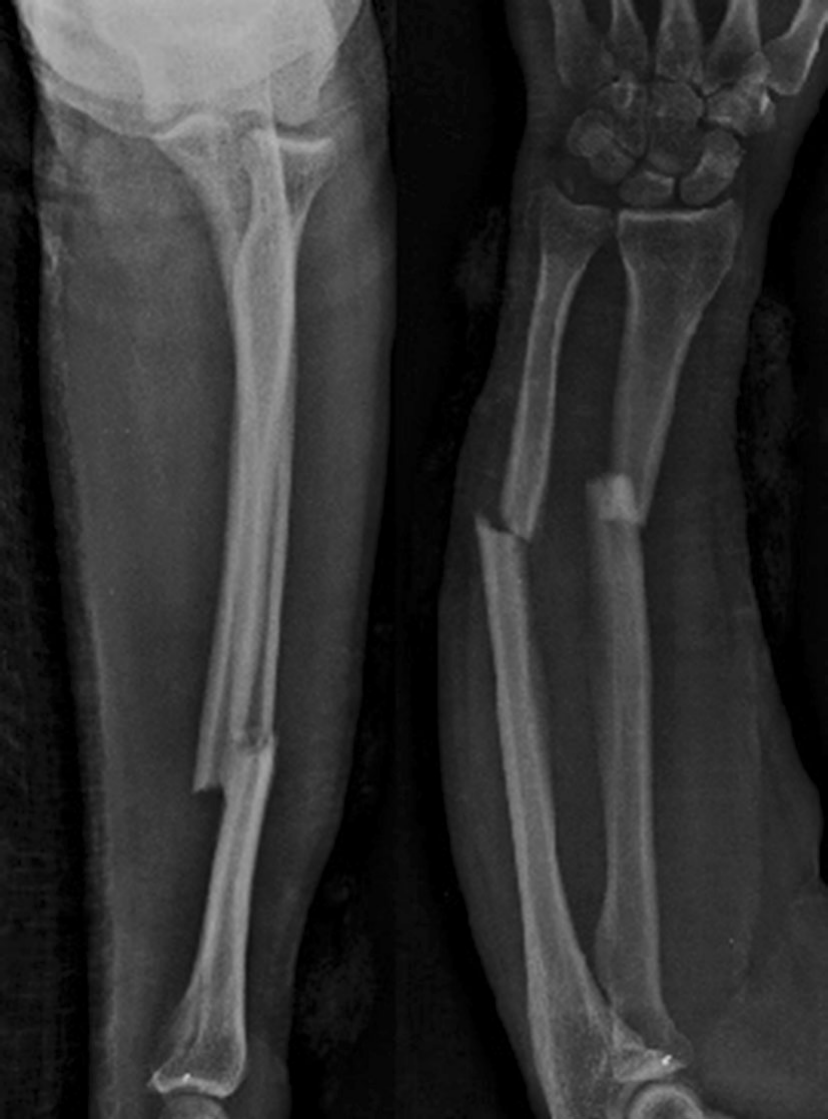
Thirty-two-year-old female patient, preoperative anteroposterior (AP) and lateral direct radiograph of the AO/ASIF Type 22A3 displaced fracture following a fall
